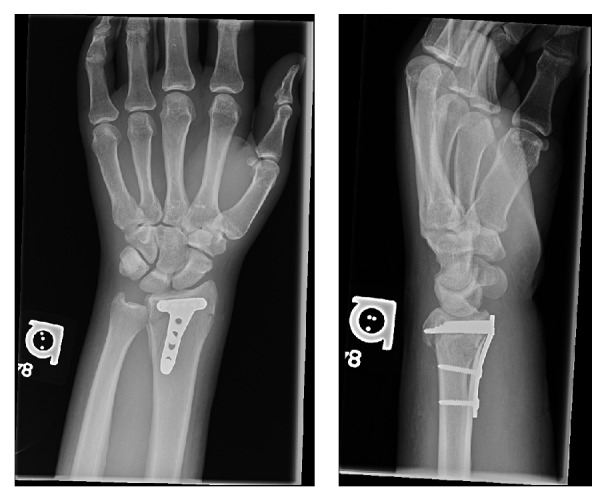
Anteroposterior and lateral X-rays of the affected wrist at the 6-week follow-up.
